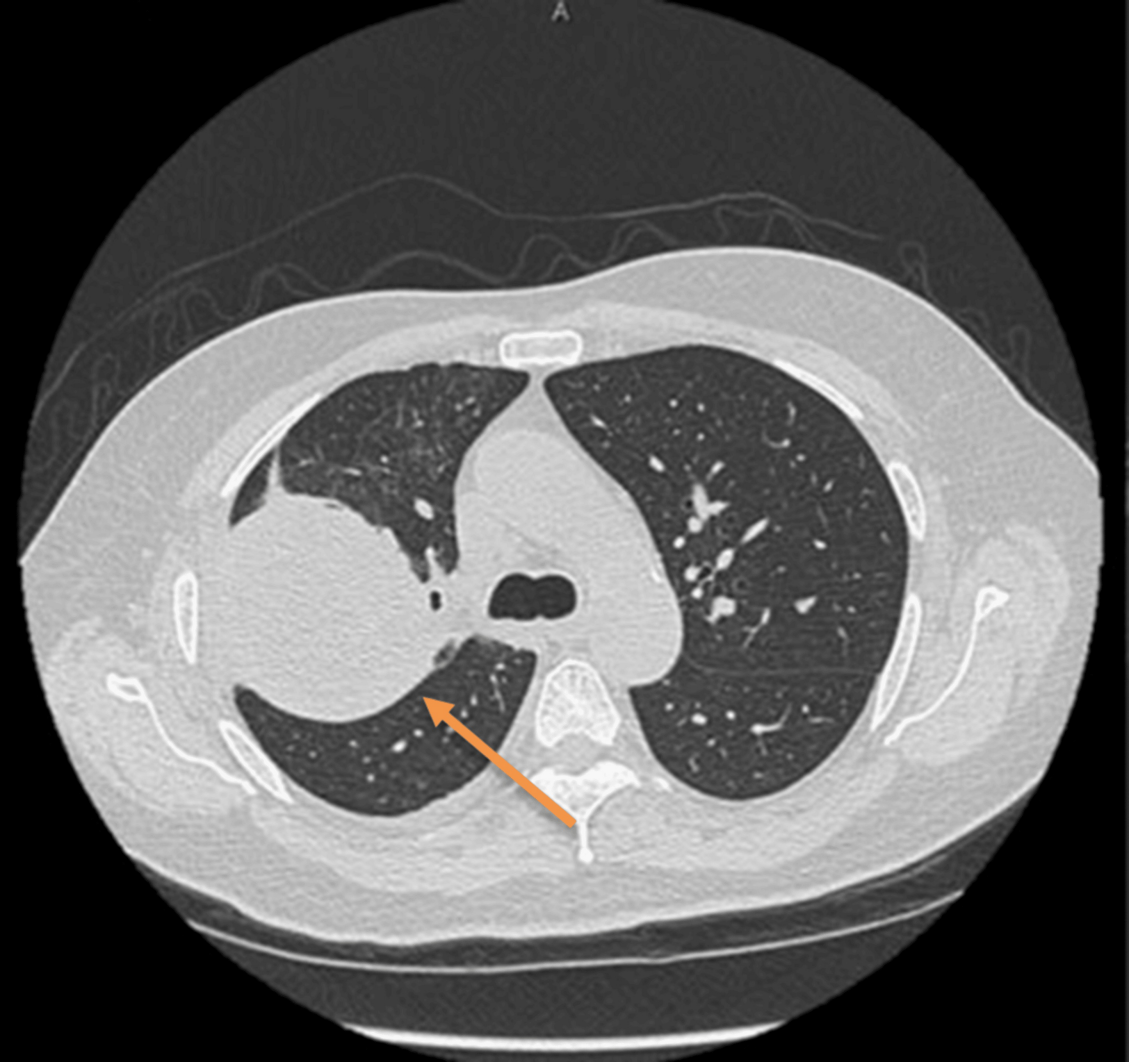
Thoracic CT imaging before lobectomy for pulmonary hydatid cyst with aspergilloma. Follow-up thoracic computed tomography (CT) scan performed two months after bronchoscopy showing interval progression of the right upper lobe cavitary lesion, now measuring 8 × 7 cm with multiple internal air loculi. Newly developed patchy parenchymal shadowing is seen in the apical segment of the right upper lobe. Trace right pleural effusion and reactive pleural thickening are also noted.
